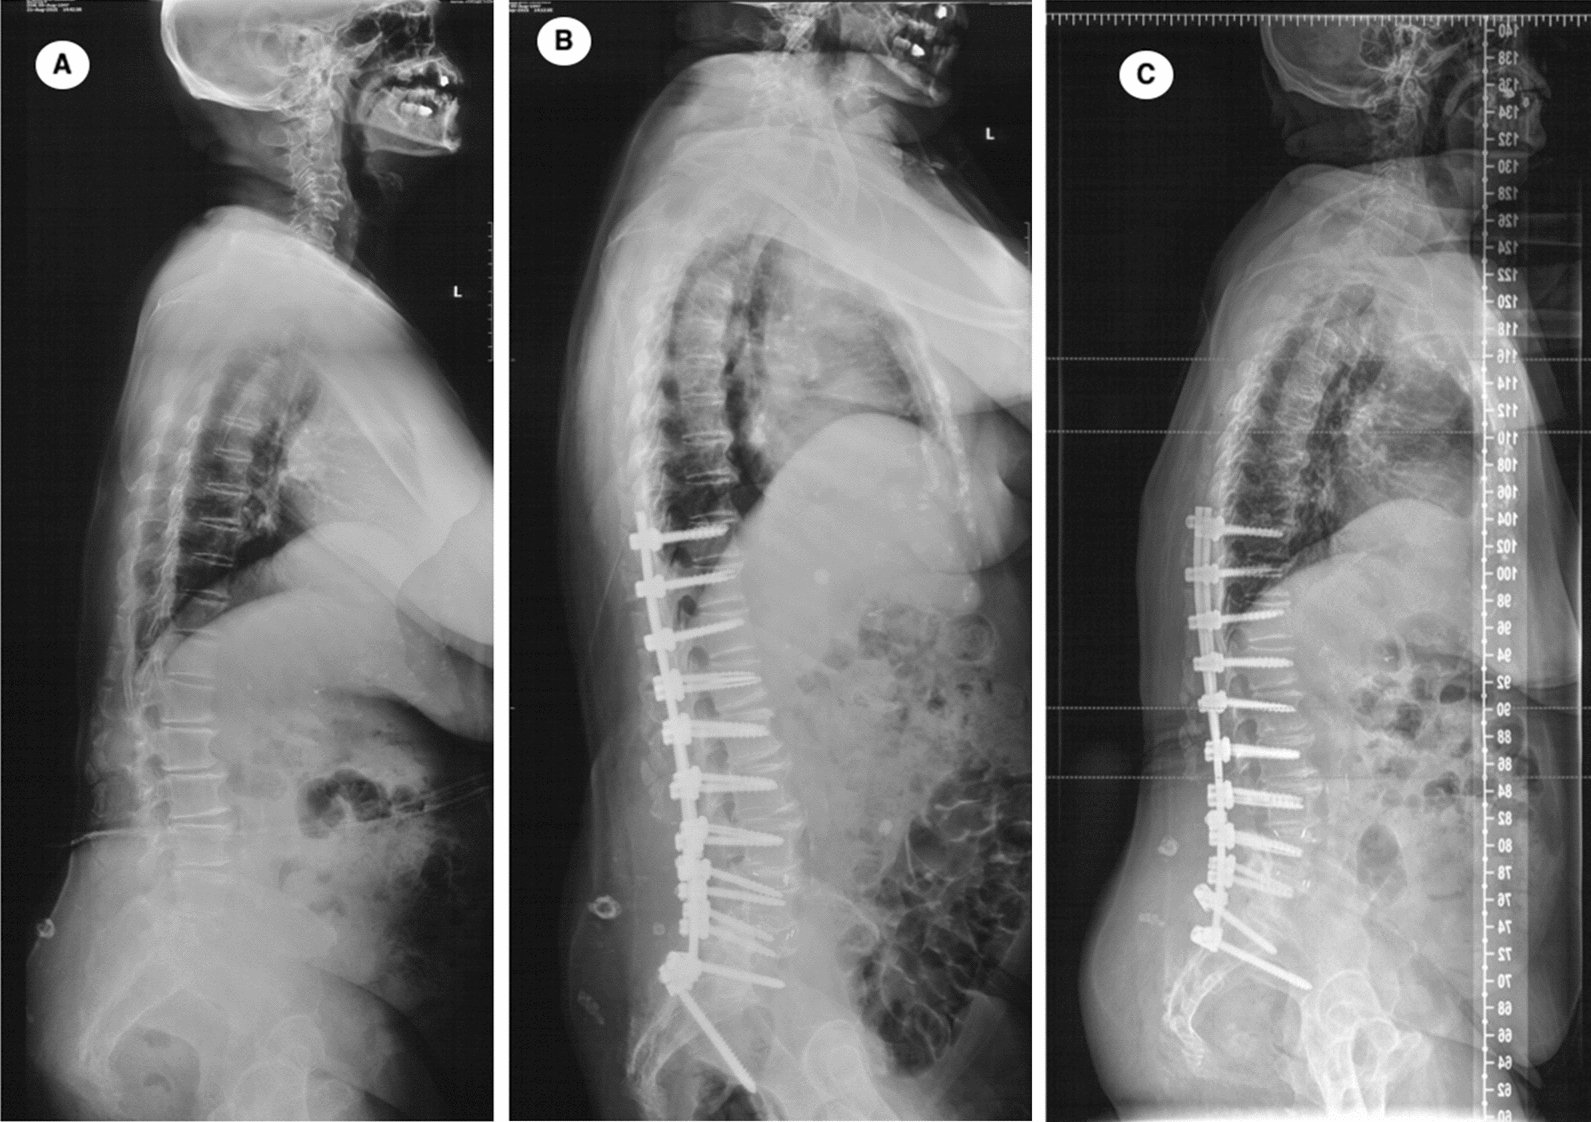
A 68-year-old female DLS patient underwent thoracolumbar fusion (T10–S2) surgery. Radiographs show the changes in spinopelvic parameters, preoperative TK, LL, PT, SS, PI, PFA, SAA, SVA and TPA were 11.3°, −7.3°, 27.3°, 20.9°, 48.2°, 206.4°, 52.0°, 90.7 mm and 29°, respectively (A). Postoperatively, those variables were 20.3° for TK, −25.1° for LL, 24.7° for PT, 21.8° for SS, 49.5° for PI, 193.8° for PFA, 50.3° for SAA, 15.3 mm for SVA, and 26.2° for TPA (B). The PI–LL was 24.8° at the post-operation. However, the patient had intermittent back pain after surgery, and PJK developed at the 4^th^ month during follow-up (C)
